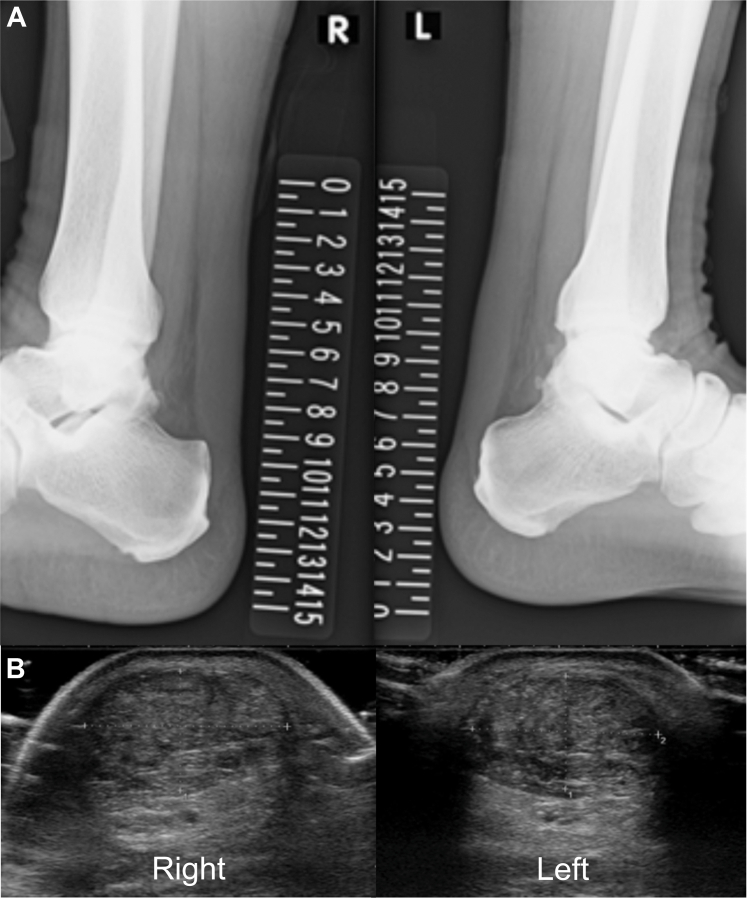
Structural Imaging of Achilles Tendons in the 35-Year-Old Son (A) Lateral ankle radiography showing increased soft tissue density consistent with Achilles tendon thickening. (B) Ultrasonography revealing marked bilateral thickening (right: 11.8 mm, left: 11.2 mm), heterogeneous echotexture, and hyperechoic foci.
